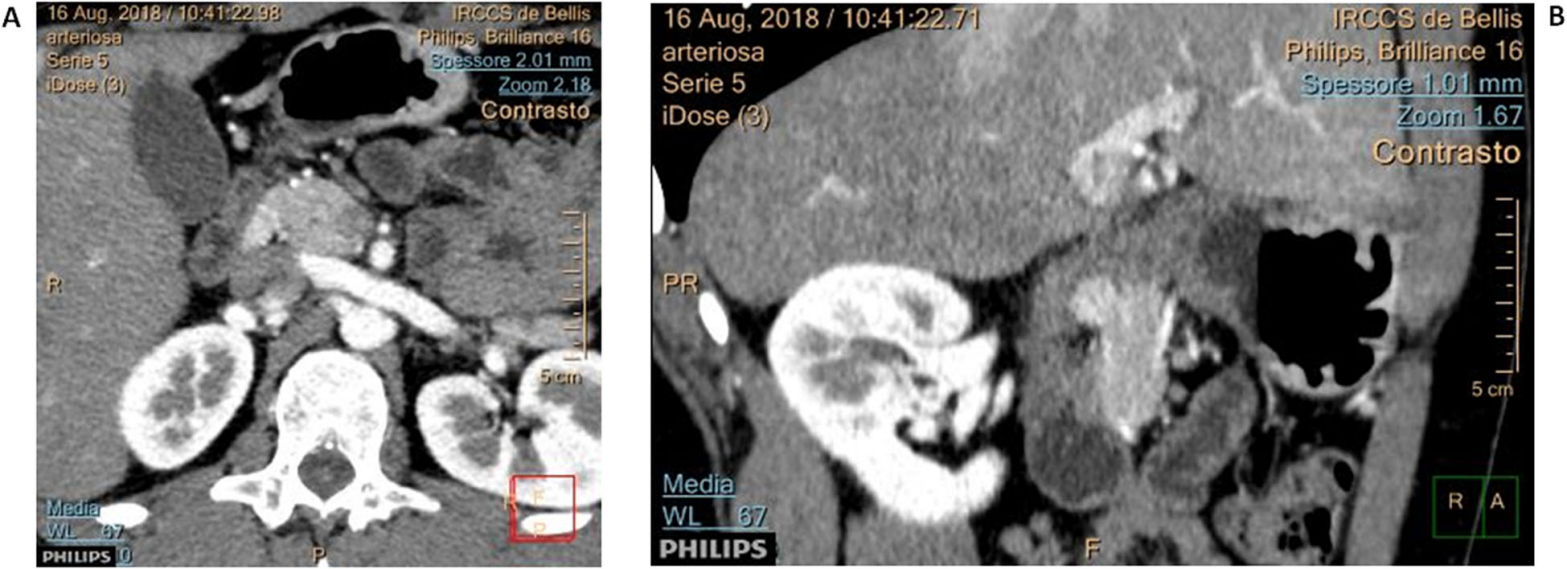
Computed tomography (CT) scan of the abdomen. a, b Axial sections from abdominal contrast CT demonstrating a 13 mm lesion (arrow) adjacent to the second part of the duodenum, corresponding to an area of abnormal octreotide uptake
